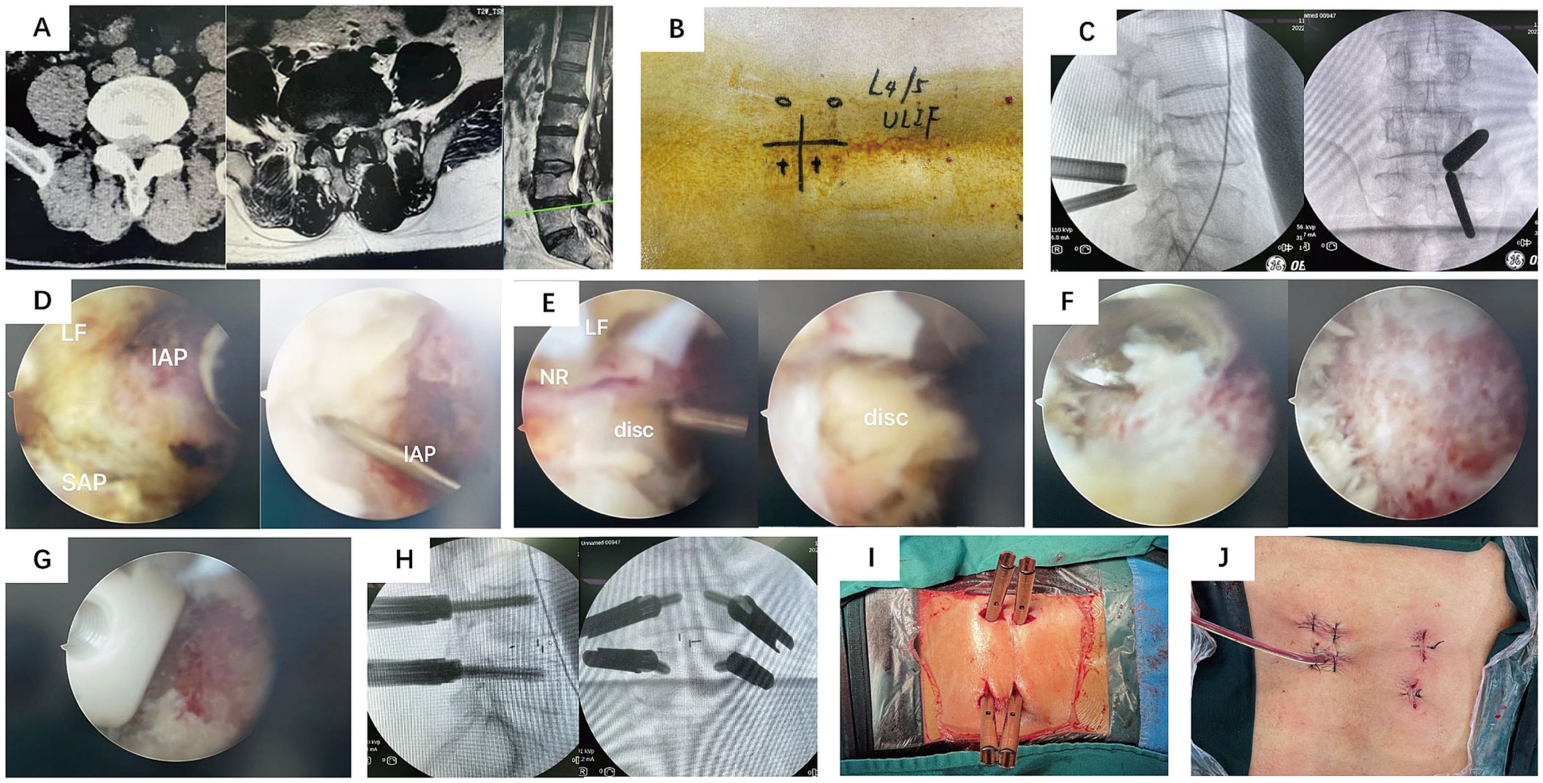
Representative Case 1. A 54-year-old male with low back pain and right lower limb radicular pain for 2 years, aggravated for 4 months. (A) Preoperative CT and MRI: right-sided L4/5 lumbar disc herniation compressing the nerve root and lateral recess stenosis. (B) Gross photo of the skin incisions. (C) Intraoperative fluoroscopic image for level localization. (D–G) Intraoperative endoscopic views showing decompression and cage insertion. (H) Intraoperative fluoroscopic image after pedicle screw placement. (I,J) Postoperative photos of the surgical wounds.
